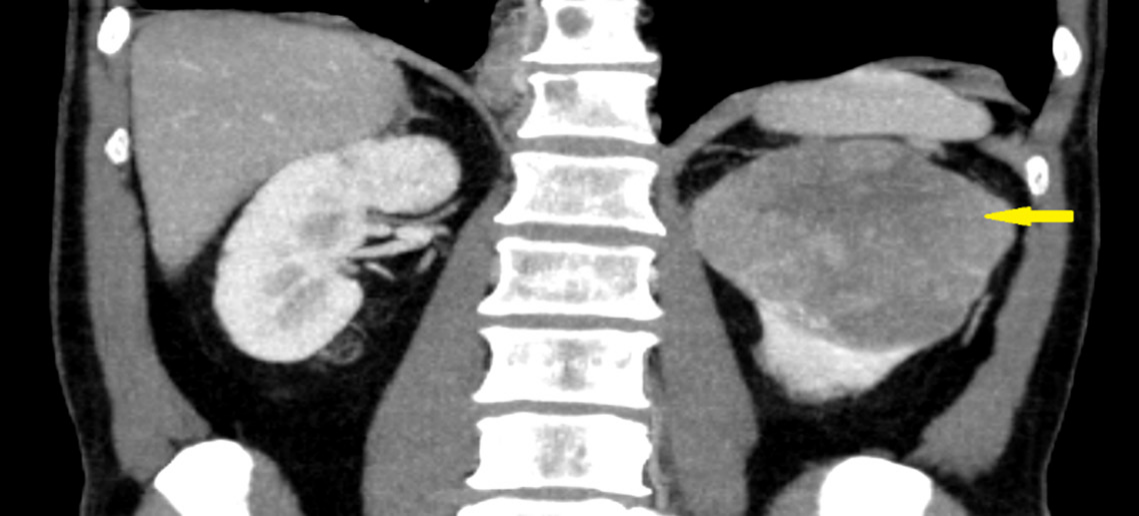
Multiple lytic destructive lesions were noted in the dorsal and lumbar vertebrae. Brain magnetic resonance imaging (MRI) showed a metastatic focus 20 × 15 mm in diameter in the right retro-orbital area (Figure 2a).
